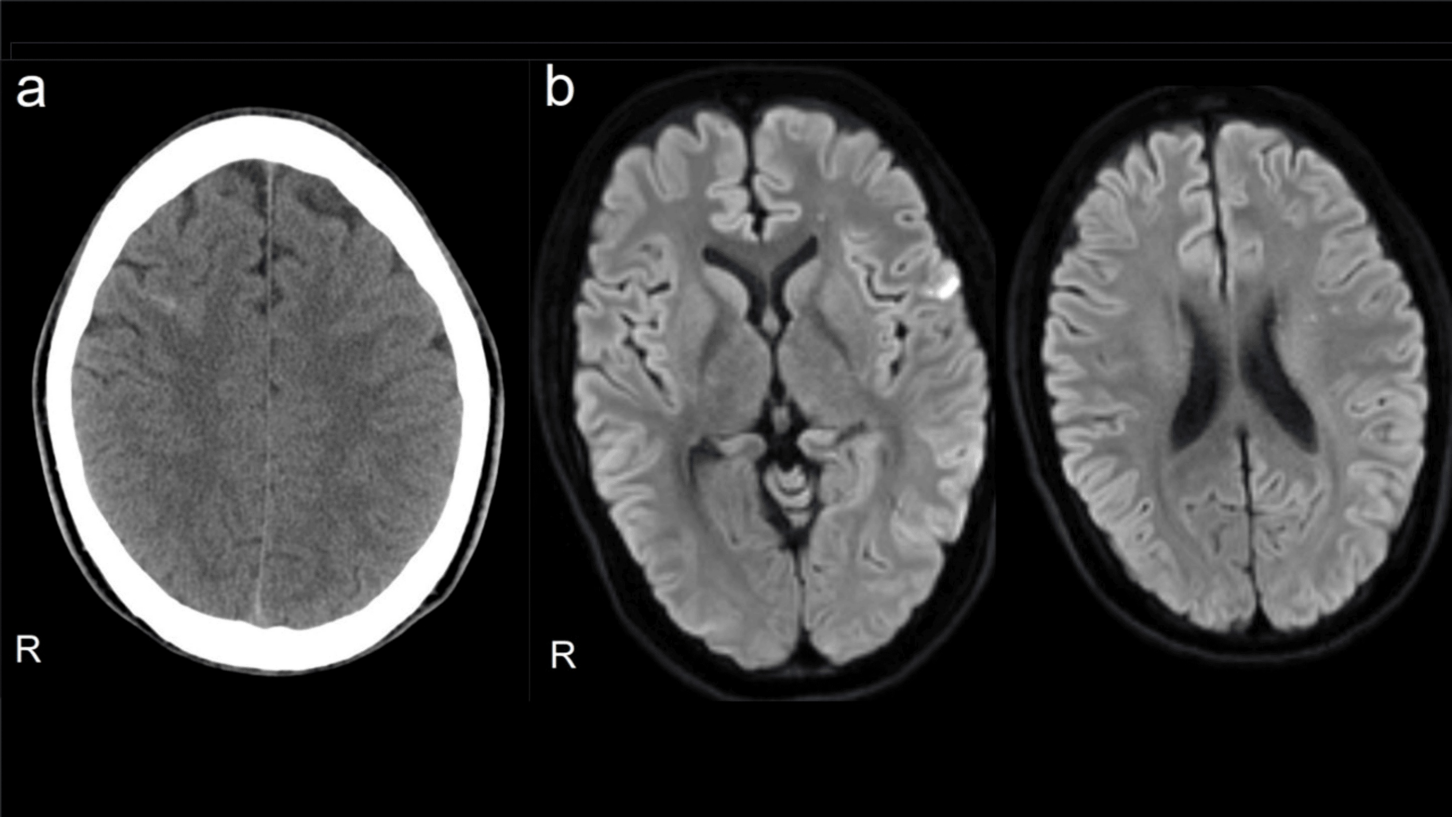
(a) Brain CT, showing SAH in the right frontal sulcus. (b) Brain MRI diffusion-weighted image, showing hyperintensity areas that appear in the left frontal lobe CT: computed tomography; SAH: subarachnoid hemorrhage; MRI: magnetic resonance imaging
